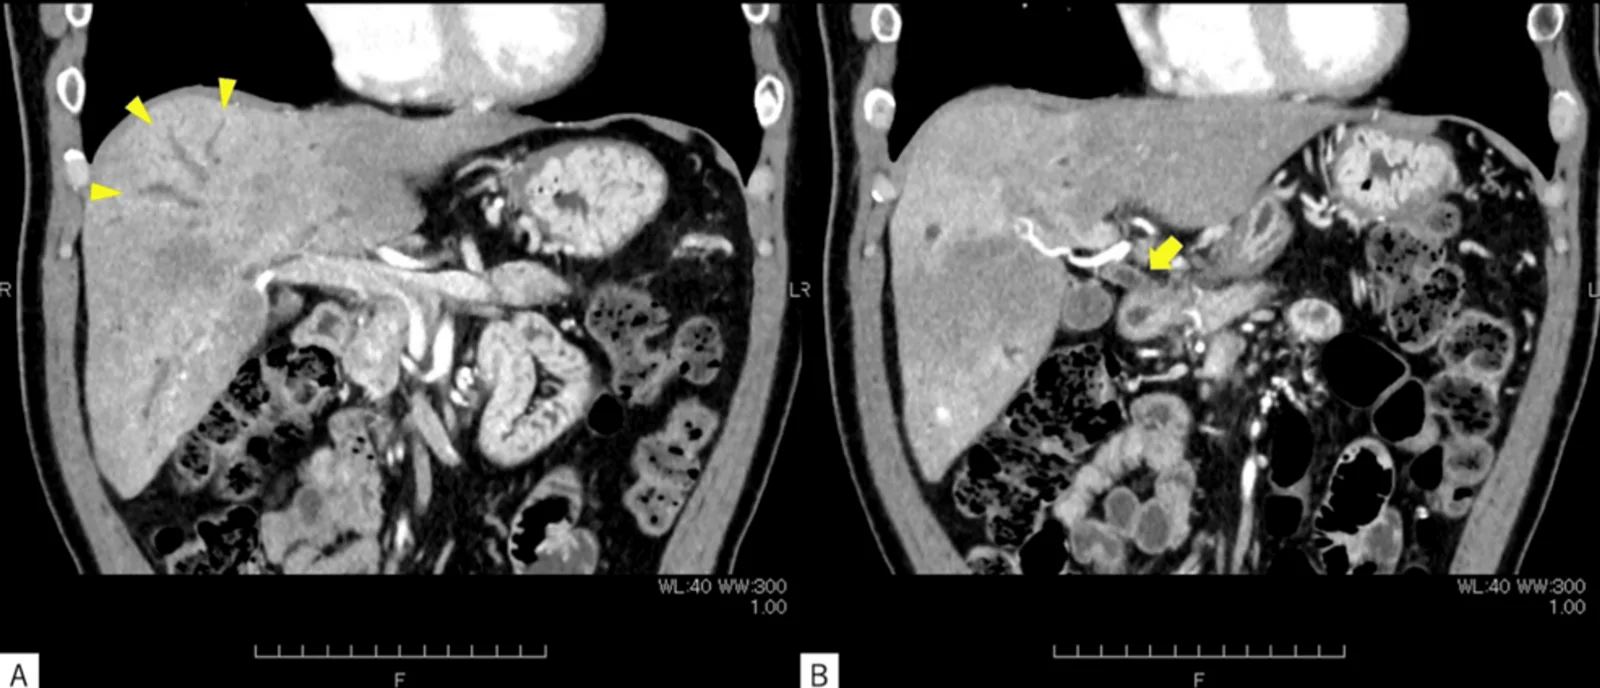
Contrast-enhanced CT findings at the time of relapse (Month 32) (A) Coronal arterial-phase image demonstrating multiple dilations of the peripheral intrahepatic bile ducts (arrowheads). (B) A different coronal section in the same phase shows diffuse wall thickening of the common bile duct (arrow) CT: computed tomography
